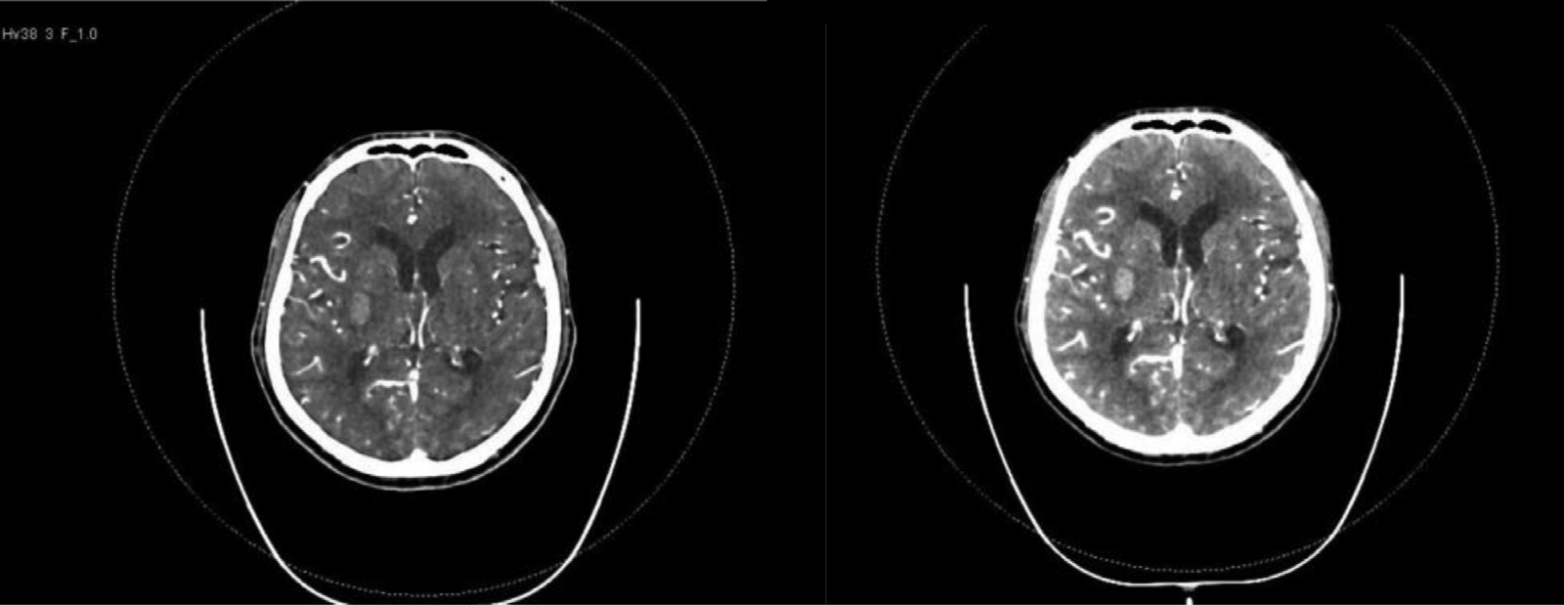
CT intracranial angiography (CTA) findings: atheromatous calcified plaques were noted at bilateral internal carotid arteries. Bilateral internal carotids, MCAs and ACAs, show adequate caliber and contrast opacification. Non-opacification for a small segment at the origin of the right vertebral artery shows diffuse narrow caliber compared to the left. The terminal part of the left vertebral artery shows a small segment of significant stenosis. The basilar artery and bilateral PCAs appear grossly unremarkable. No evidence of intracranial aneurysm or vascular malformation.
